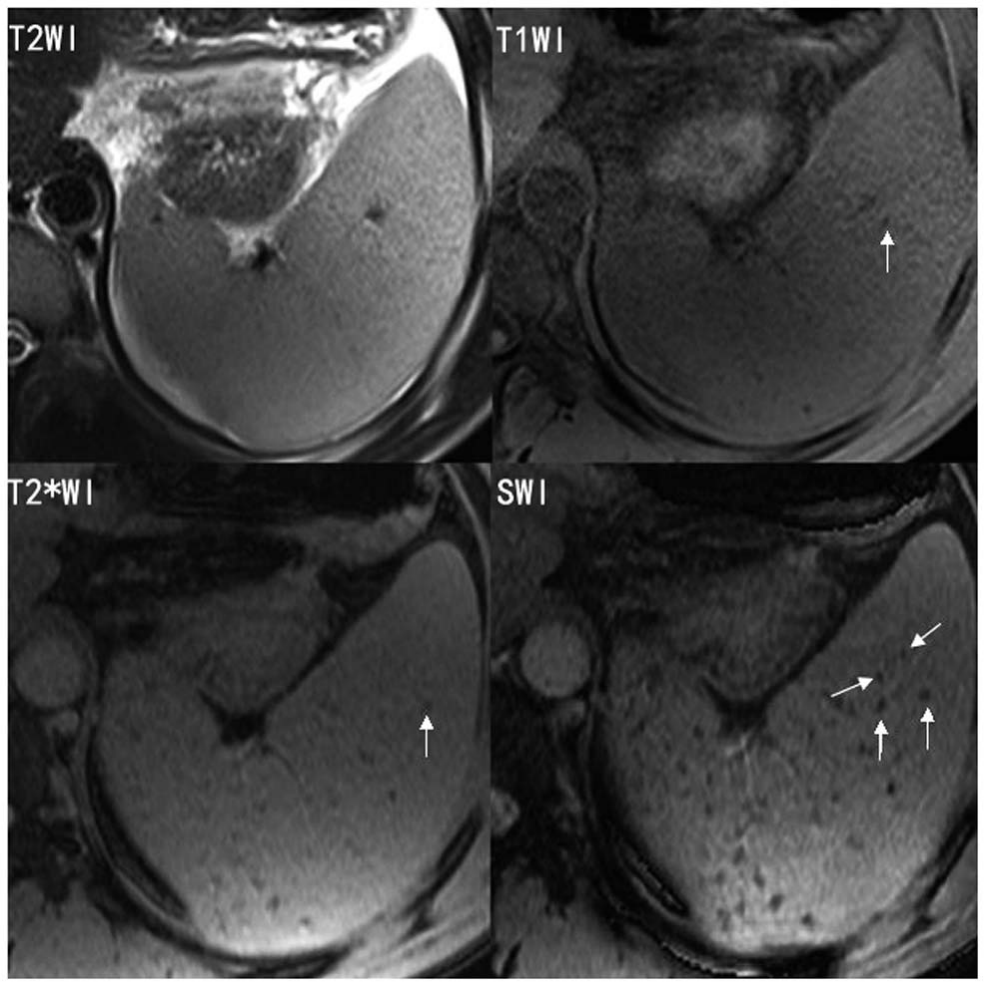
Representative T1-weighted image (T1WI), T2-weighted image (T2WI), T2*-weighted image (T2*WI) and susceptibility-weighted imaging (SWI) of a 42 year-old patient with splenic siderotic nodules. SWI shows more siderotic nodules than T1WI, T2WI or T2*WI.
